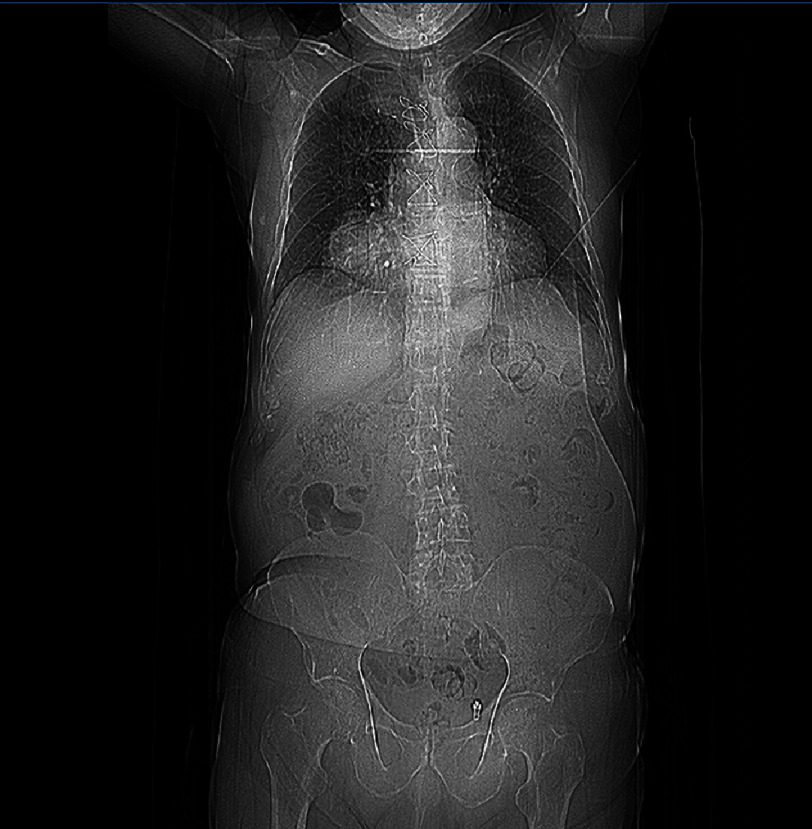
Chest X-ray showing an enlargement of the right cardiac silhouette.
